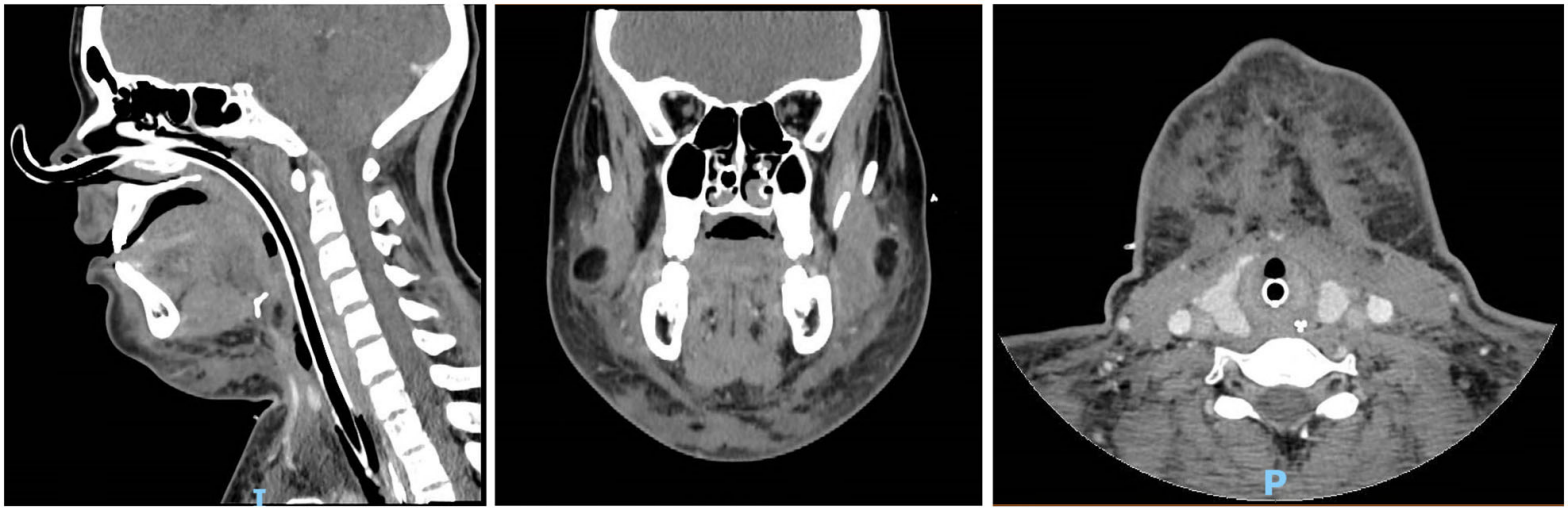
CT scan four weeks postop.
